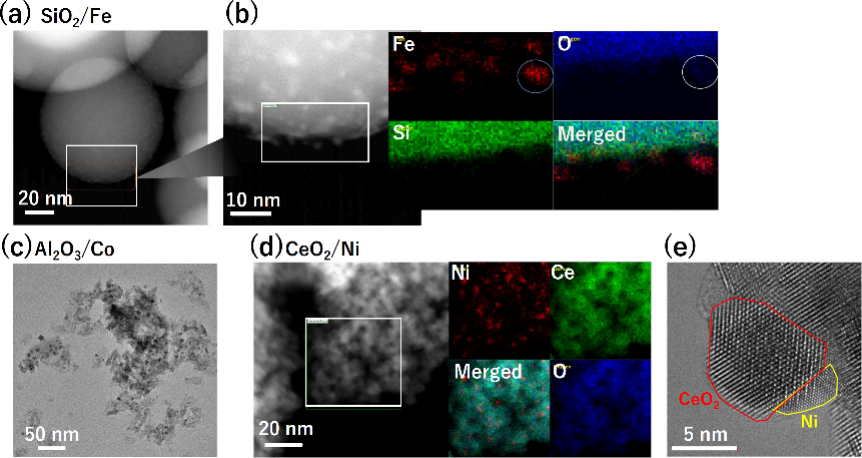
Scanning TEM (STEM) and energy-dispersive X-ray
